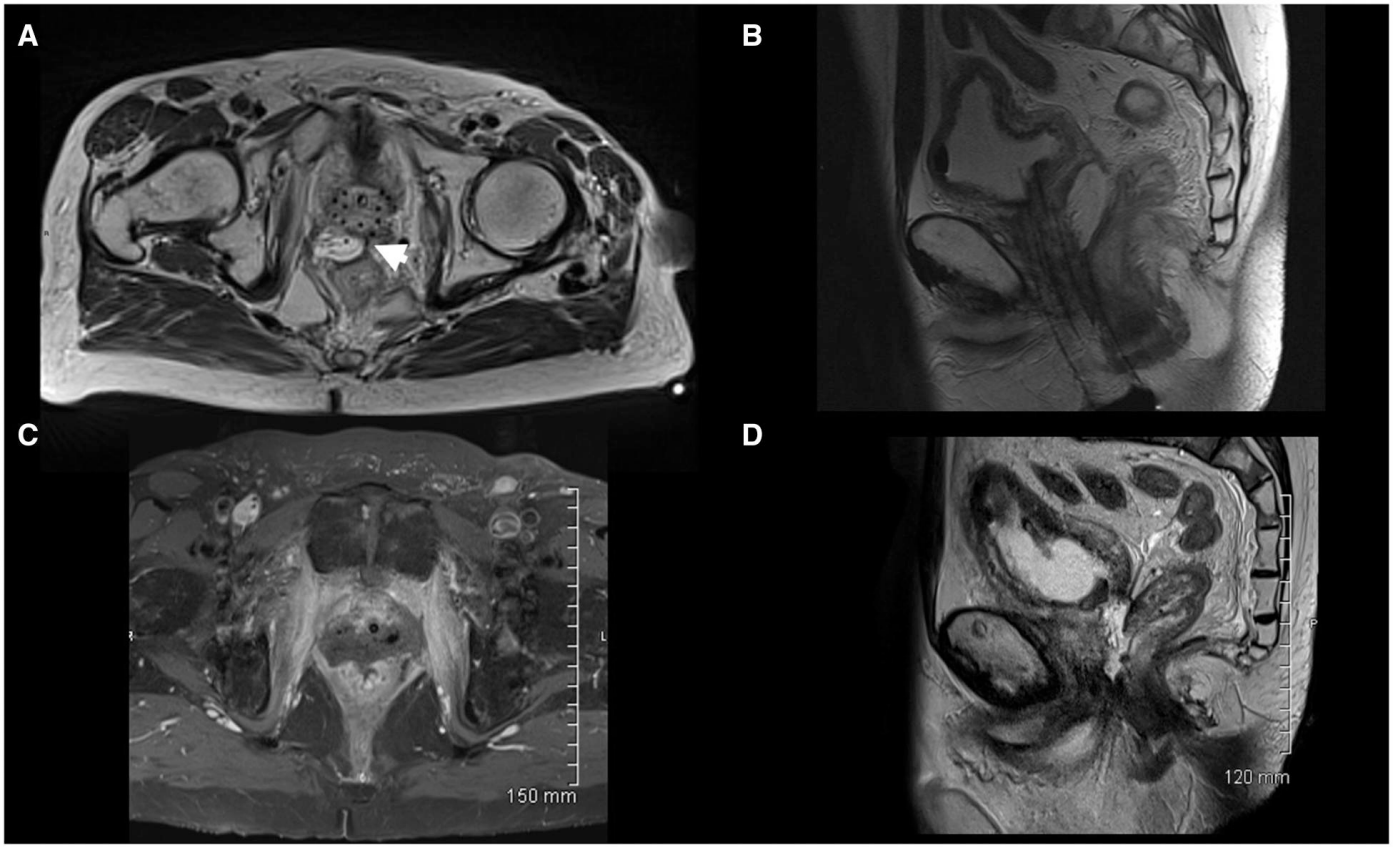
(A and B) Selected axial and sagittal images from a T2 weighted MRI pelvis completed after insertion of the brachytherapy wires showing the hydrogel spacer to be positioned between the rectum and the prostate. The spacer has an asymmetrical distribution with more of the hydrogel on the right. A small adhesion is seen between the prostate and the rectum (arrow head). (C and D) Selected axial T1 weighted images with intravenous contrast and T2 weighted images from an MRI pelvis completed 1 month after brachytherapy was completed. These images show a recto-urethral fistula. There is a defect in the anterior rectal wall communicating with a collection between the rectum and the prostate. The collection is continuous with the prostatic urethra (urinary catheter in situ).
